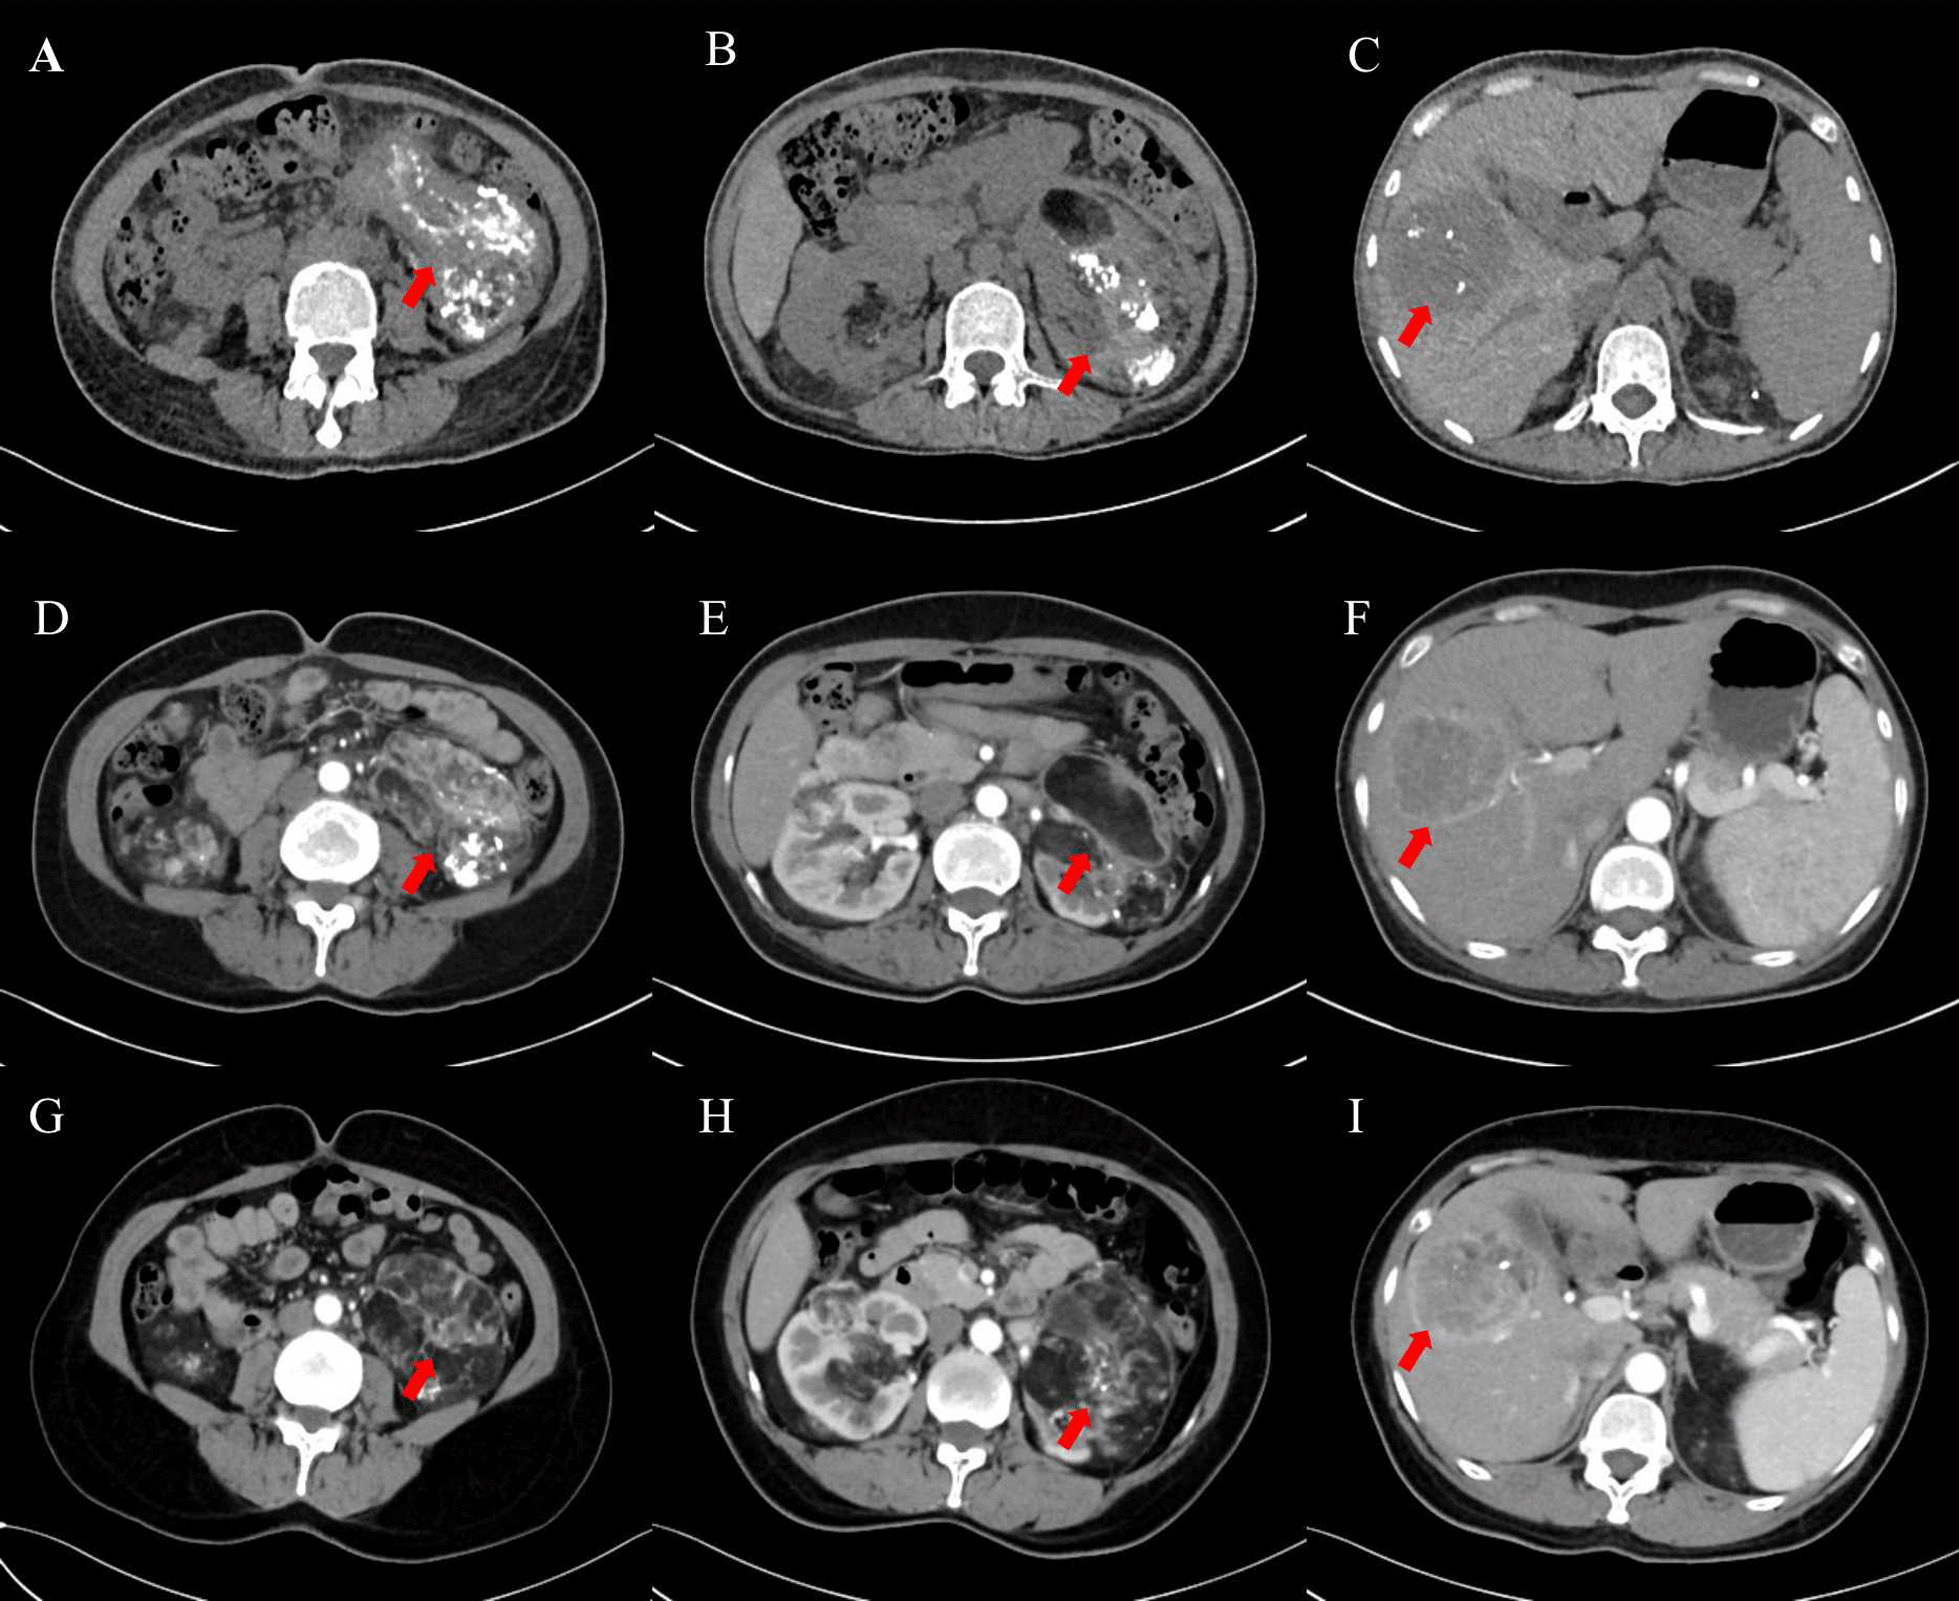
CT images of the patient during follow-up. A–C CT images obtained at 4-month follow-up. D–F CT images obtained at 6-month follow-up. G–I CT images obtained at 18-month follow-up. The arrows in A, B, D, E represent kidney lesion locations. The arrows in C, F represents the location of the liver lesion
